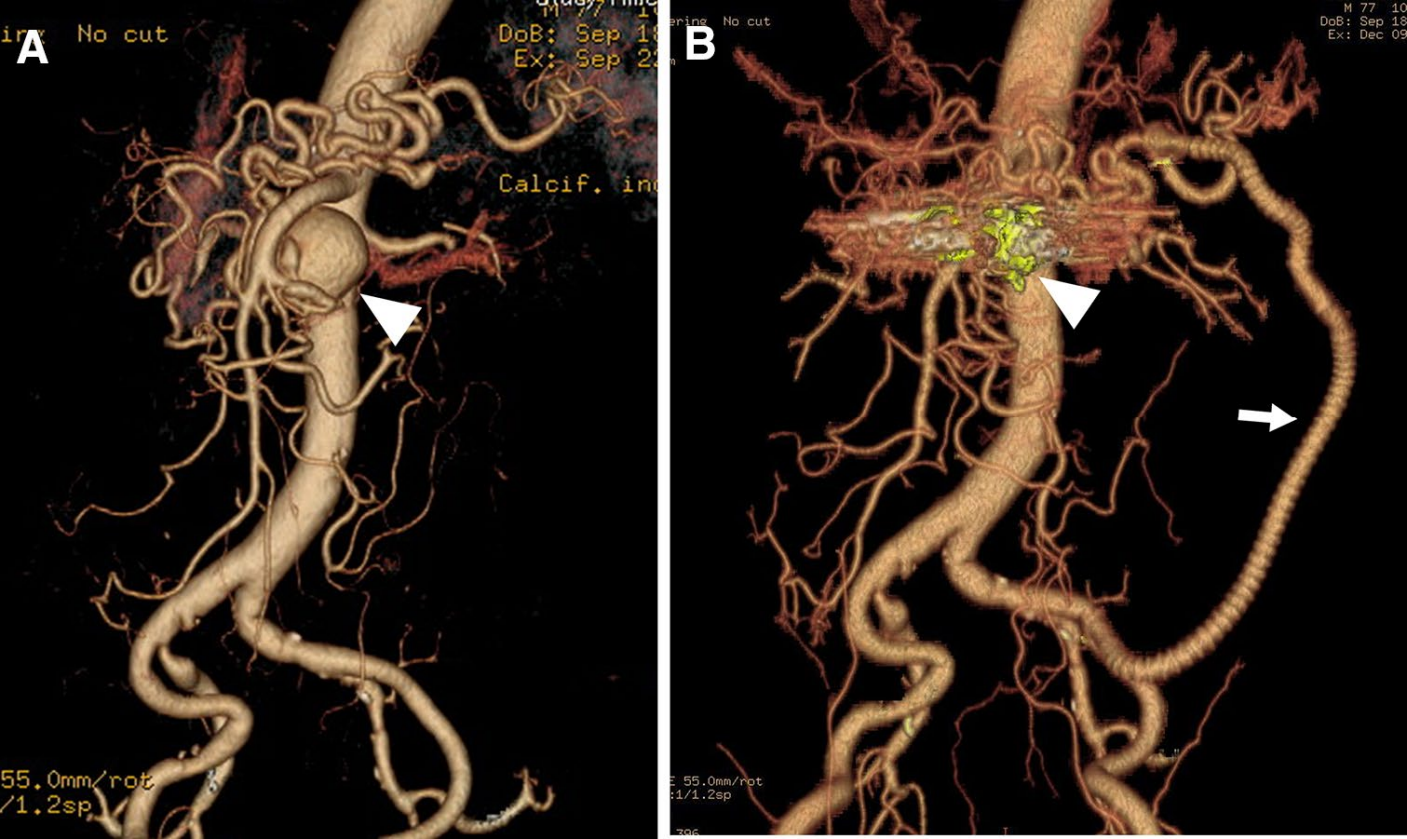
Inferior pancreaticoduodenal artery aneurysm in 77-year-old man. a Three-dimensional volume rendered computed tomography (CT) angiography shows a saccular aneurysm (35 mm) of the inferior pancreaticoduodenal artery (IPDA), adjacent to the origin of the IPDA with celiac axis occlusion. The main blood flow to the liver and spleen was supplied via this IPDA aneurysm. b Postoperative three-dimensional volume rendered CT angiography shows the successful coil embolization (arrowhead) and the patent prosthetic graft (arrow) from the iliac artery to the dorsal pancreatic artery to preserve organ blood flow
